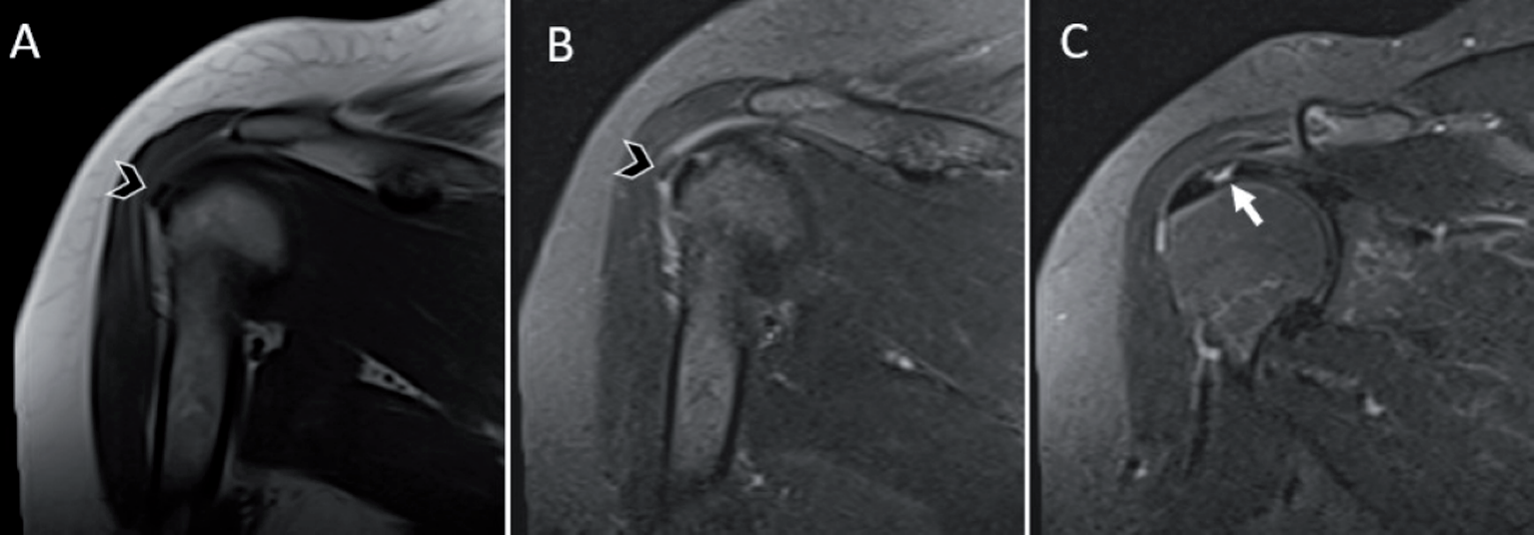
Calcific tendinitis associated with a tear at a different location within the same tendon. Right shoulder images of a 60-year-old female patient.(A)Coronal T1-weighted and (B)coronal proton density–weighted magnetic resonance imaging (MRI) images demonstrate an approximately 7 mm calcific focus at the humeral insertion of the supraspinatus tendon (arrowhead).(C)A different coronal T1-weighted MRI image shows an approximately 5-mm articular-sided partial-thickness tear of the supraspinatus tendon (arrow).
